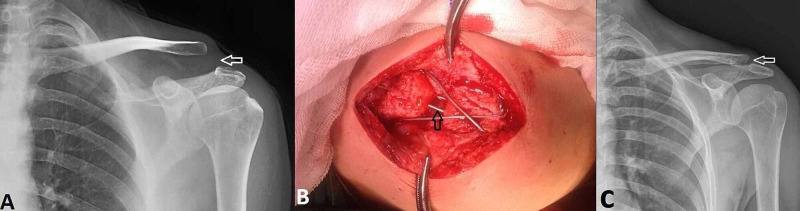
Patient with Rockwood type III acromioclavicular joint dislocation (A) Anteroposterior radiographic images of the joint show a dislocation of the acromioclavicular joint of the left shoulder (white arrow). (B) Intraoperative view of the acromioclavicular joint after reduction and fixation with tension band wiring technique (black arrow). (C) Radiographic image of the patient's left acromioclavicular joint 3 years after surgery (white arrow).
